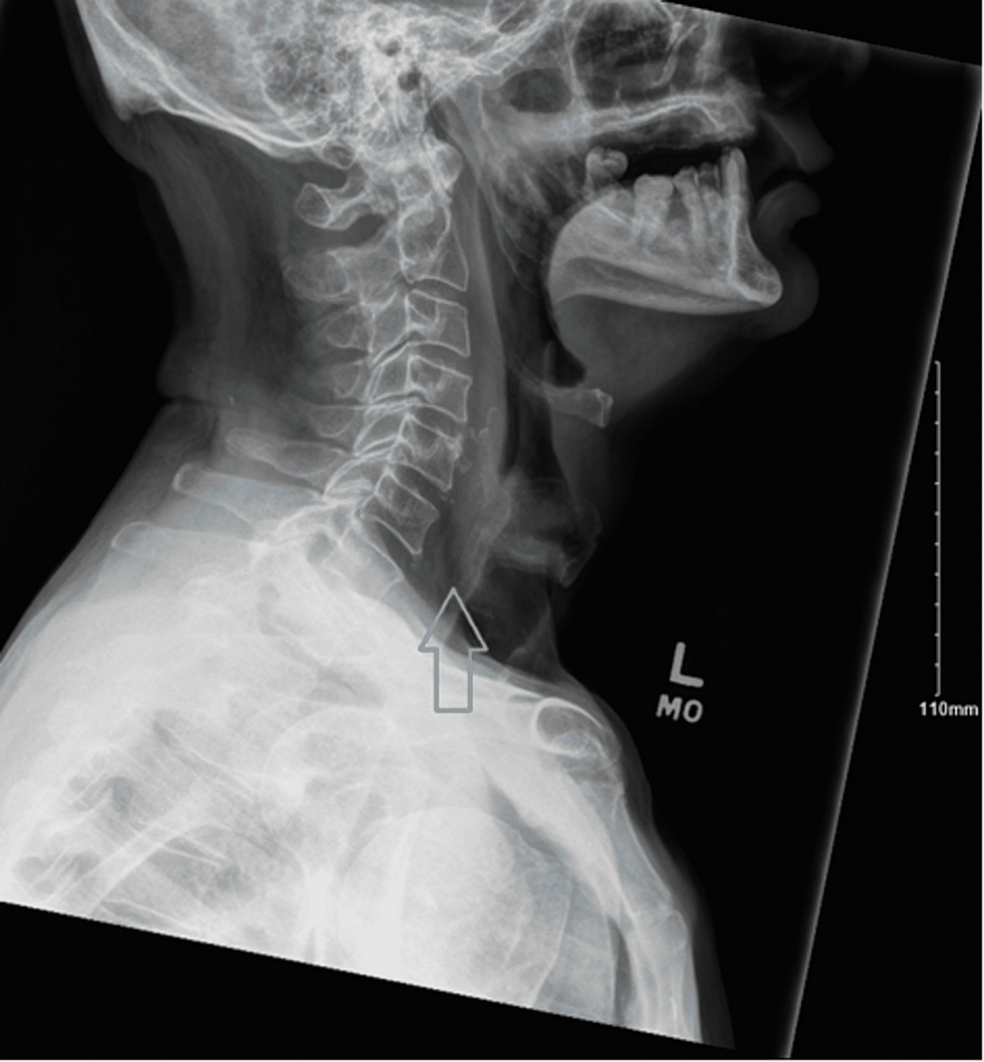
X-ray soft tissue neck revealing mild prevertebral soft tissue swelling at the level of C6 (grey arrow).
